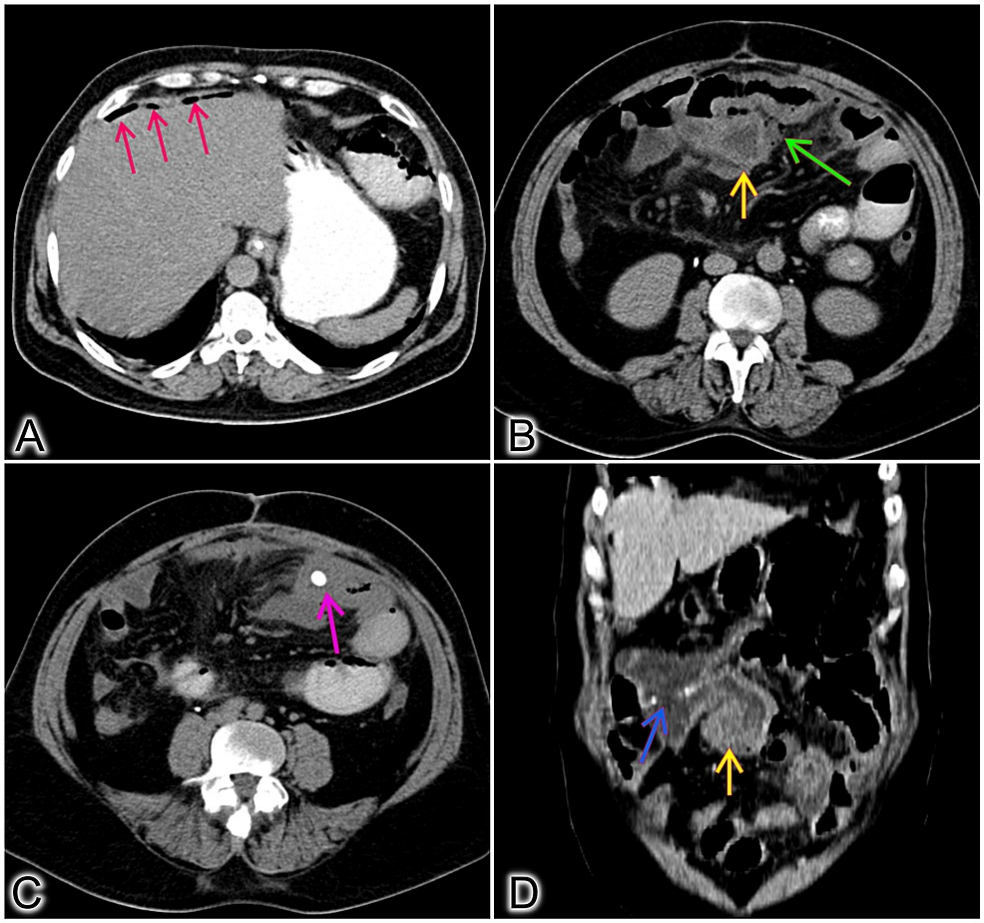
CT images. (A) Red arrows showing specs of intra-abdominal air. (B) Yellow arrow shows the thickened inflamed blind ileal loop, green arrow shows extra-luminal air with collection adjacent to the blind loop. (C) Enterolith seen in the colonic blind loop portion. (D) Coronal section showing the thickened ileal loop (yellow arrow) and the ileo-transverse lateral anastomosis (blue arrow).
